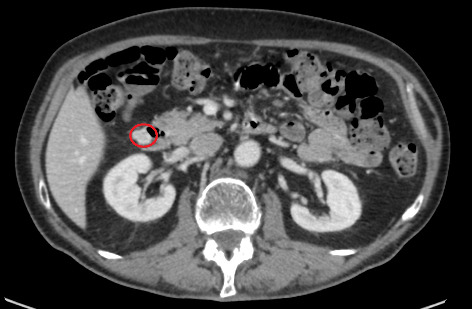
Axial abdominal intravenous contrast-enhanced computed tomography of the duodenal tumour (red circle). Helsinki University Hospital, Diagnostic Centre, Radiology, Helsinki, Finland.
